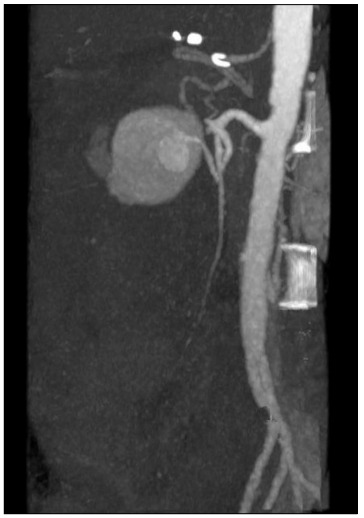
Preoperative computed tomography scan (three-dimensional) showing aneurysmatic rupture.
